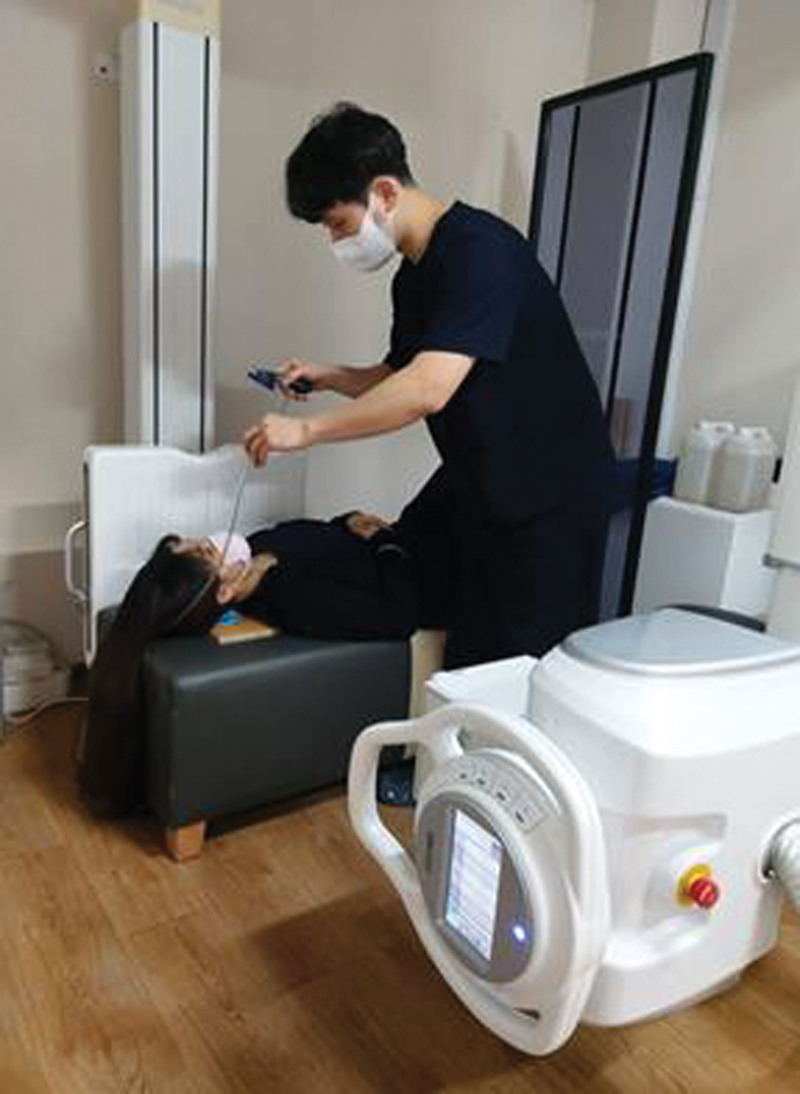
Radiographic imaging of the lateral aspect of the cervical spine during craniocervical flexion test at low stage (20–24 mm Hg).
